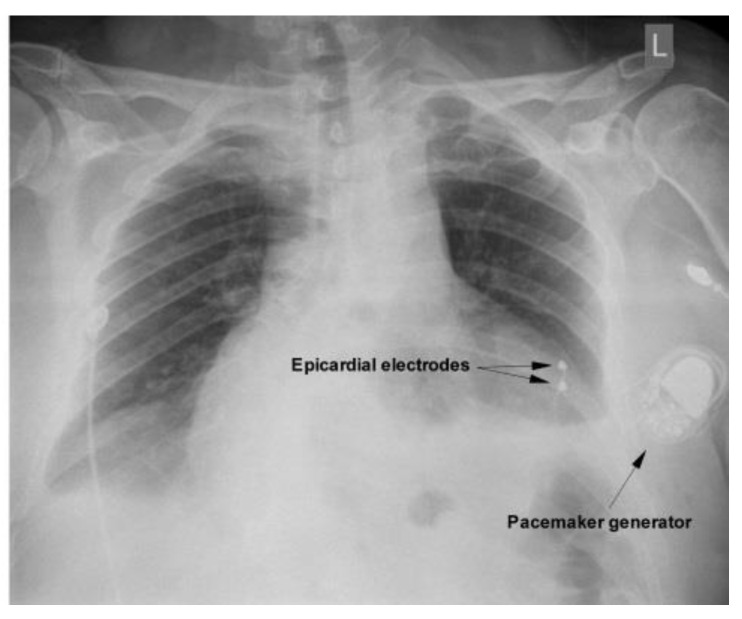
Follow-up chest radiography after pacemaker implantation.
